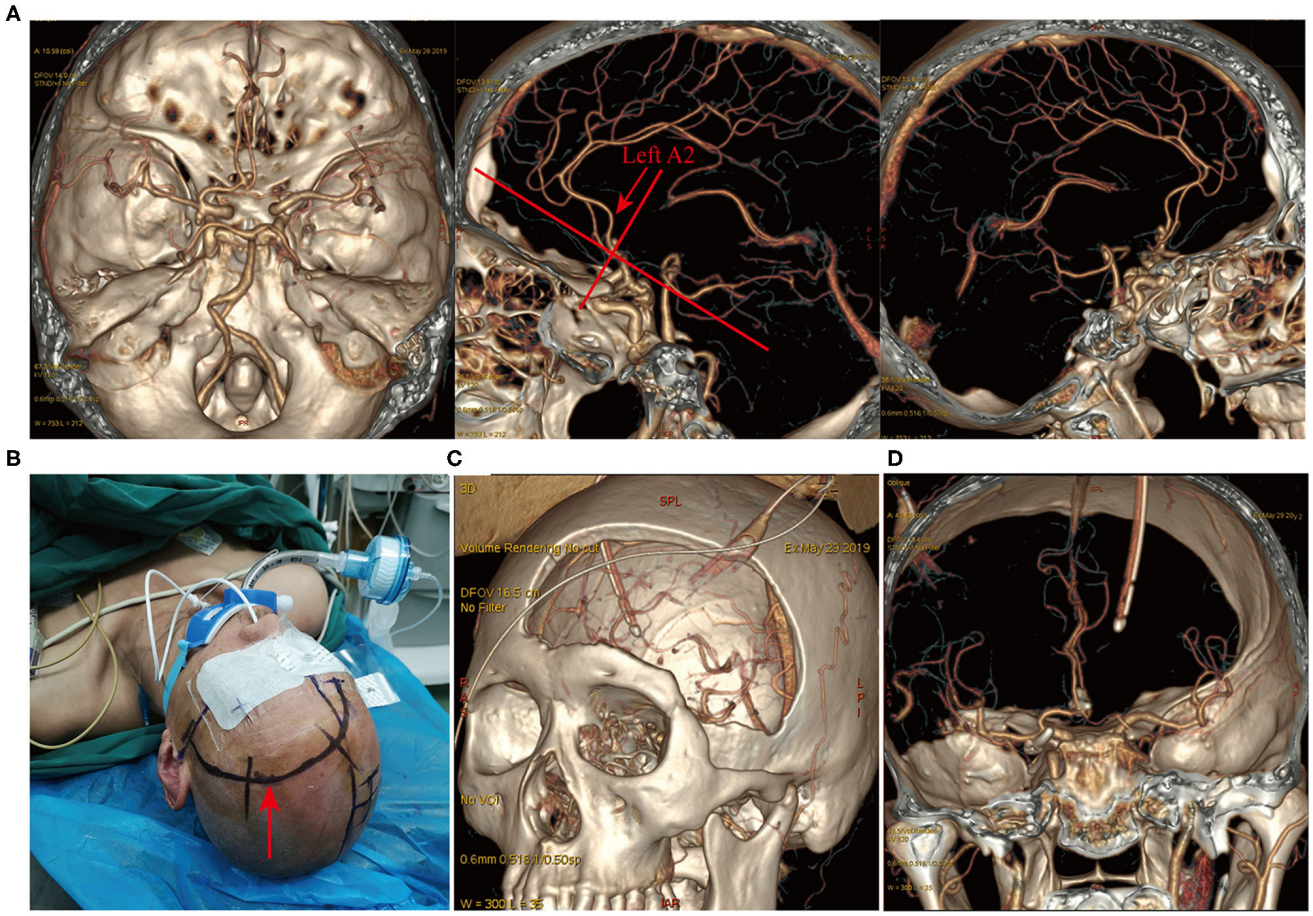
Longitudinal fissure pterion surgical approach. A patient with ruptured multiple intracranial aneurysms and hemorrhage (Hunt-Hess grade IV). (A) CTA revealed a left posterior communicating artery aneurysm and an AcoA aneurysm. Lateral view showed a posteriorly projecting AcoA aneurysm and the left A2 posterior to the right A2 (indicated by the red arrow). The left longitudinal fissure pterional approach was selected for surgery. (B) Surgical incision and patient positioning. (C) Postoperative three-dimensional CT reconstruction indicated the size and location of the surgical bone window. (D) Postoperative CTA revealed complete clipping of the AcoA aneurysm and the left posterior communicating artery aneurysm, with no remnants and good vessel protection.
